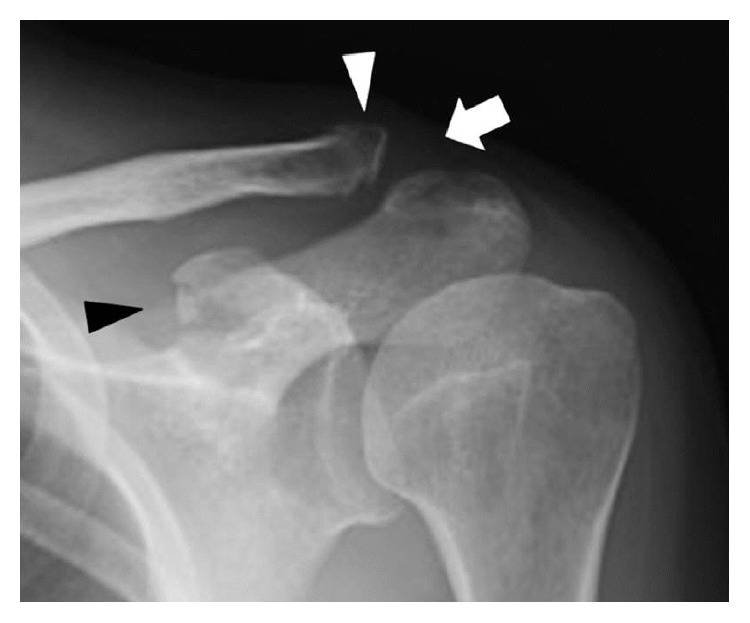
Radiograph showing an ACJ dislocation (white arrow) with distal clavicle fragment displaced upwards (white arrowhead). Upon close examination, a cortical bone discontinuity at the CP medial apex is observed (black arrowhead).
